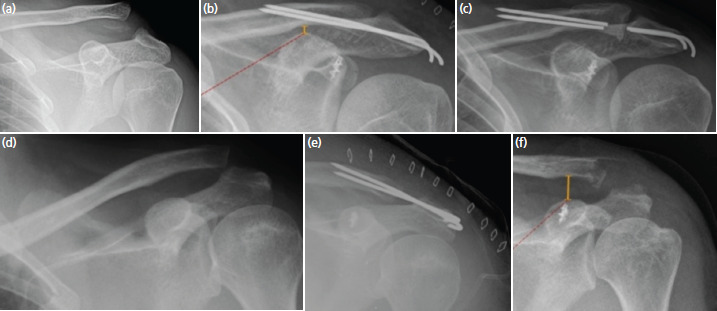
Anteroposterior radiographs of acromioclavicular joints showing post-operative complications –K wire breakage; (a) pre-op, (b) immediate post-op, (c) follow-up. Loss of reduction following K wire removal (d) pre-op, (e) immediate post-op, (f) follow-up.
